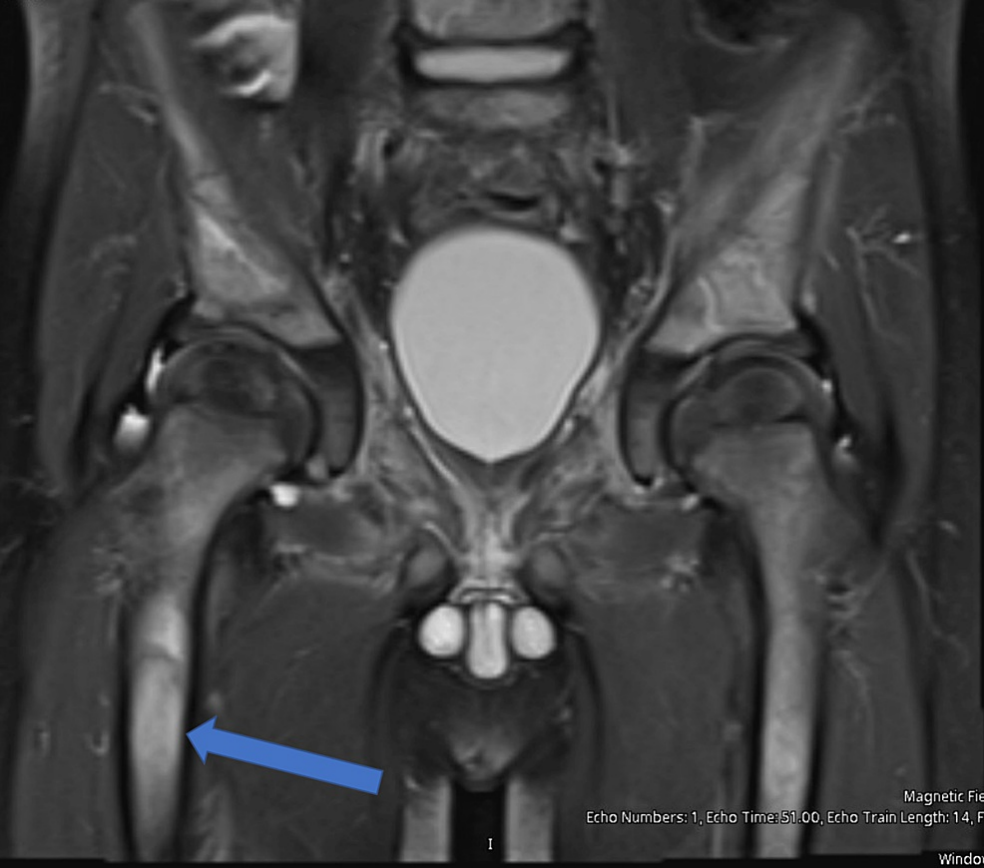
MRI of the right hip/femur demonstrating increased marrow signal on T2. The blue arrow is indicative of the increased signal seen in the more distal femur area as compared to Figure 1. T2: Transverse relaxation time, MRI: Magnetic resonance imaging
